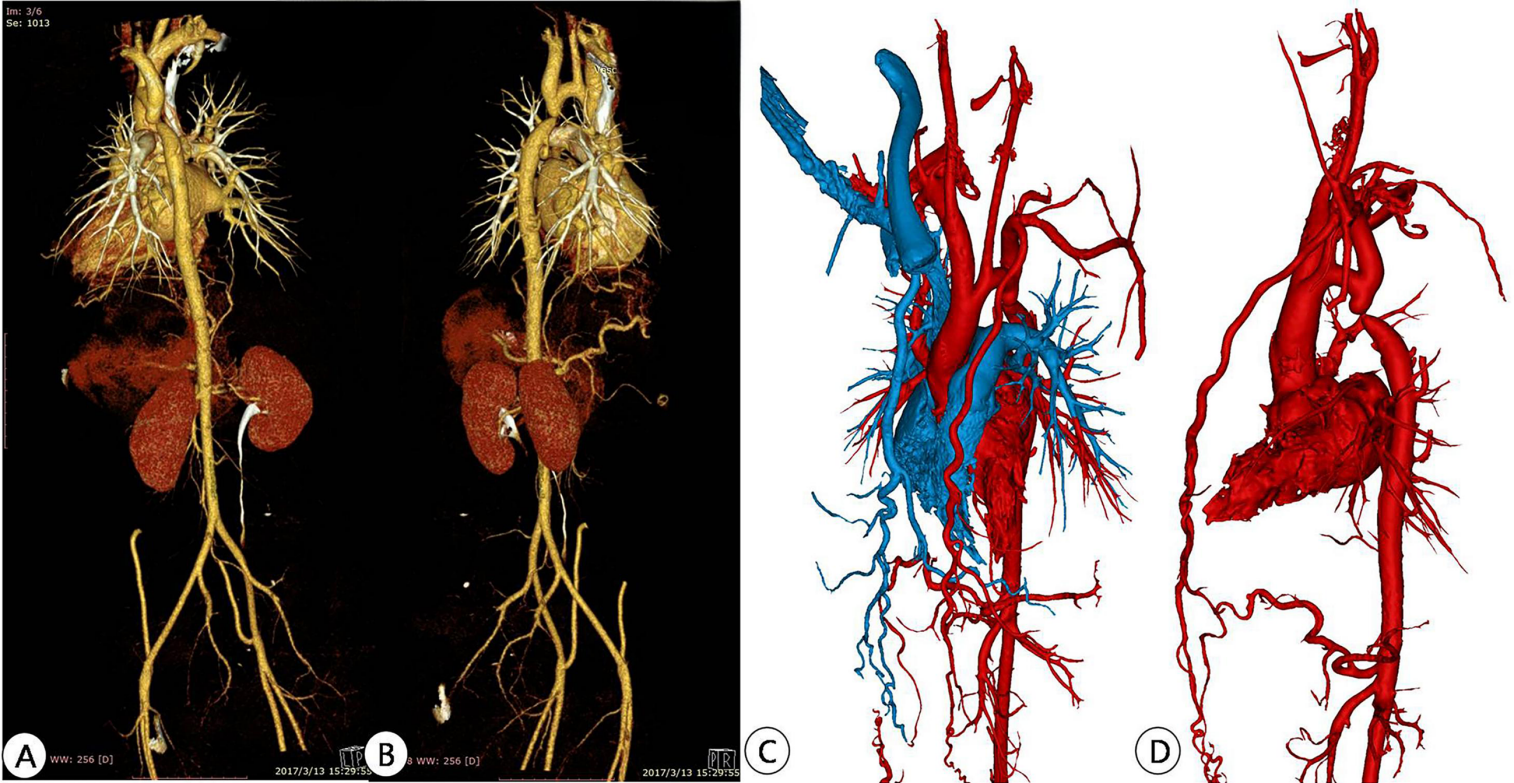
Preoperative CTA reconstruction image of the patient. (A,B) CTA reconstruction imaging shows the location of aortic arch constriction; (C,D) Using computer reconstruction technology, segment the anatomical structure around aortic constriction and highlight the location of aortic constriction.
